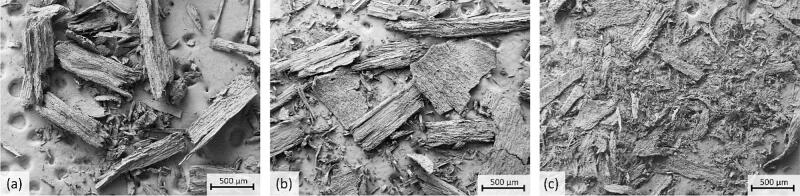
FESEM images (100x magnification) of (a) untreated sawdust, (b) sawdust (2.5 g) treated at 150–180 °C for 30 min without, and (c) with ultrasound (70:30 pulse, 3 bar).
